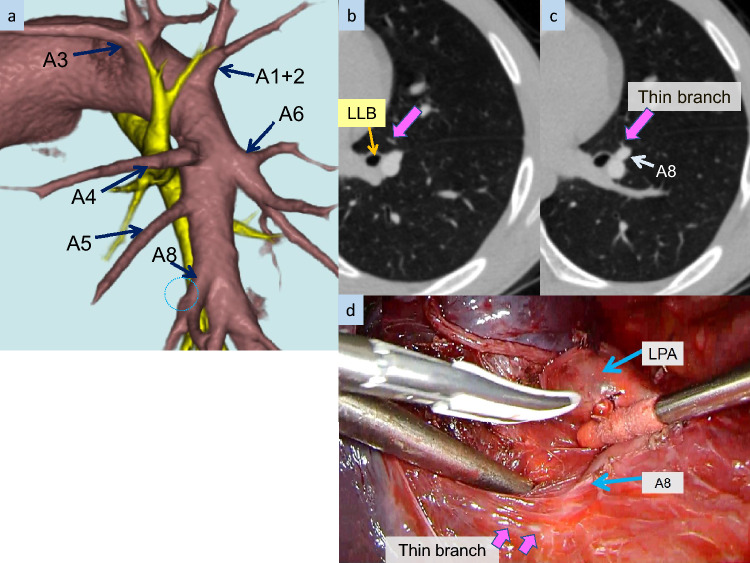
3D-CTA, thin-section CT, and intraoperative images in a male teenager suspected of having lung cancer of the left upper lobe. a–c The thin branch of the PA supplying the lingular segment branching off from A8 is depicted on thin-section CT images, although not visualized on 3D-CTA images. d The thin branch was identified intraoperatively
